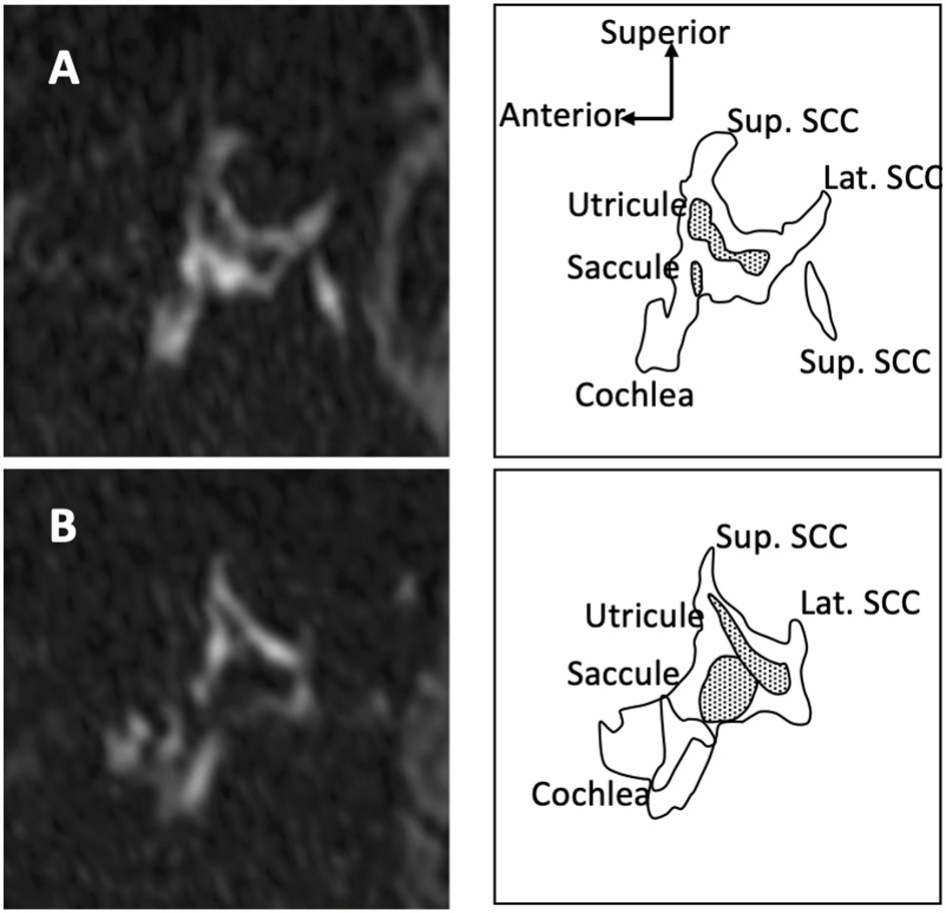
Saccule and utricle on 3D-FLAIR oblique sagittal views. When there is no hydrops (A) saccule appears smaller than utricle. In case of hydrops (B), the saccule becomes larger than the utricle and a saccule/utricle ratio inversion (SURI) can be observed.
